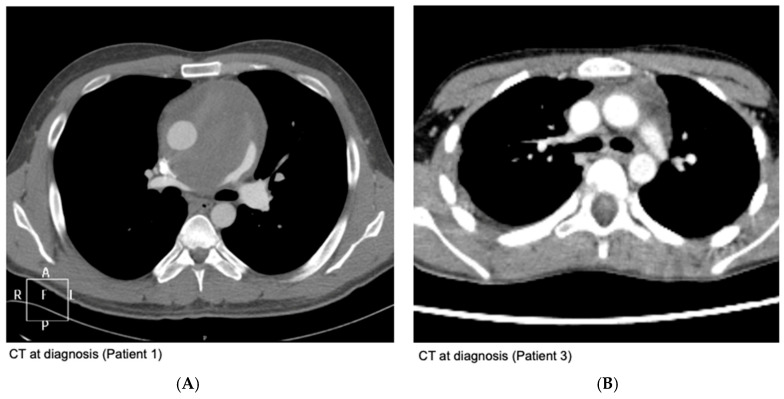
Depicts the variability in appearance of Ewing sarcoma on CT imaging. (A) Shows an unresectable tumor located in the middle mediastinum and diffusely infiltrating the heart. (B) Shows a small, localized tumor of the anterior mediastinum.
